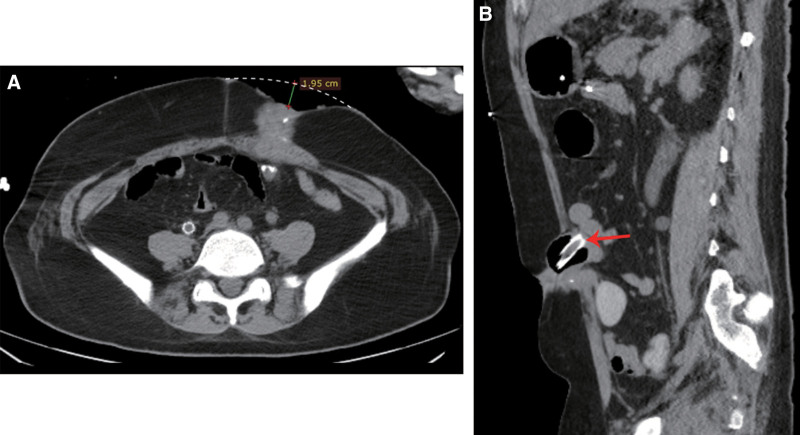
(A) Abdominal computed tomography (CT) revealed significant retraction of the sigmoid colostomy. (B) Follow-up CT revealed the modified endotracheal tube maintained excellent sealing and full intestinal wall contact. CT = computed tomography.
